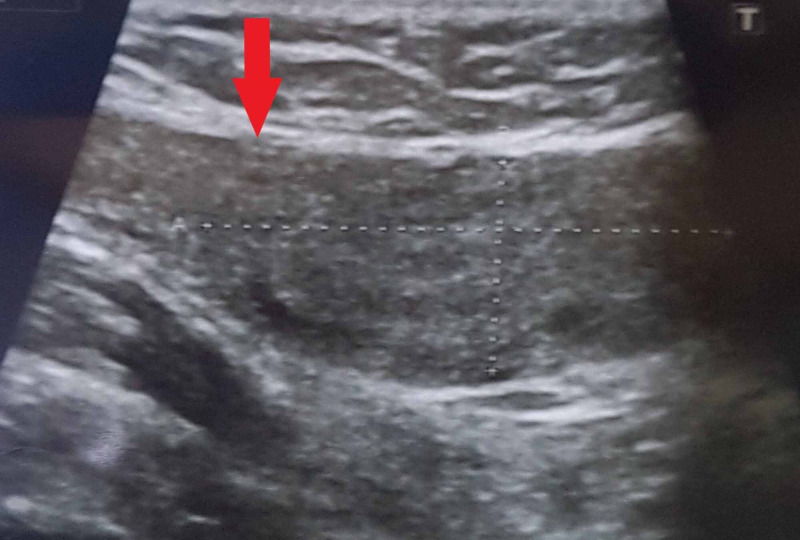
Hypoechogenic elongated nodular thickening of left vas deferens on ultrasound.
